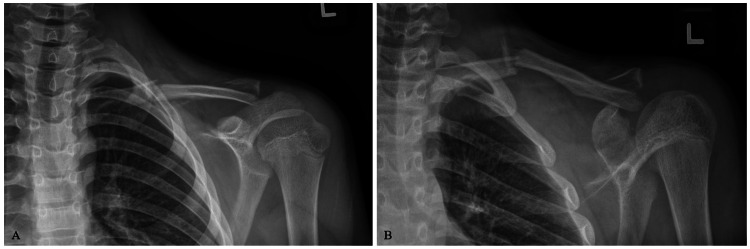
Injury radiographs, upright anteroposterior and Zanca views (A-B), demonstrating displaced, segmental clavicle fracture.
